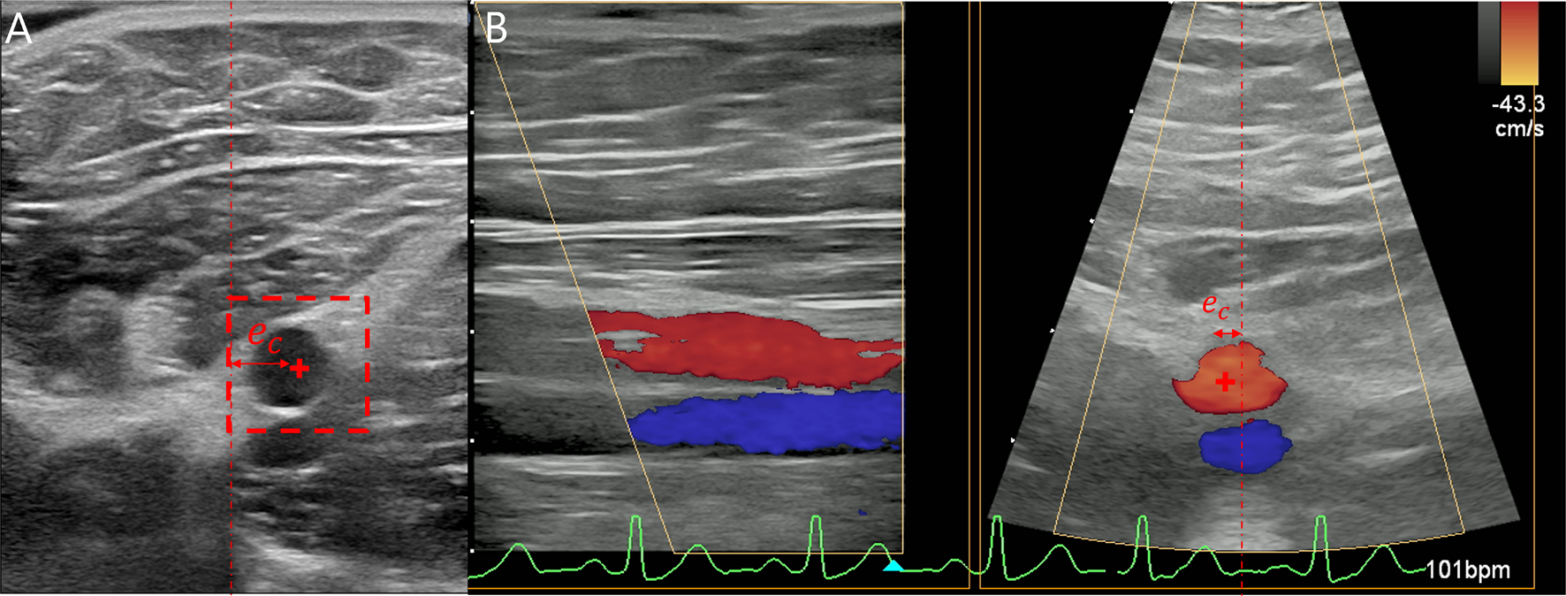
A Vessel position determined by template matching. B Determination of the vessel position from the Doppler X-Plane US image
